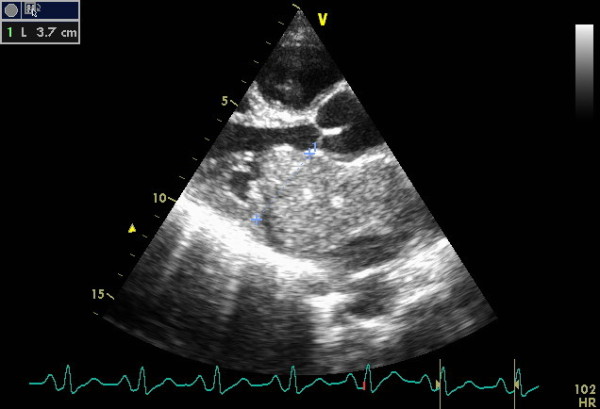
Transthoracic echocardiography long-axis view shows a giant left atrial myxoma fills almost the entire left atrium.
